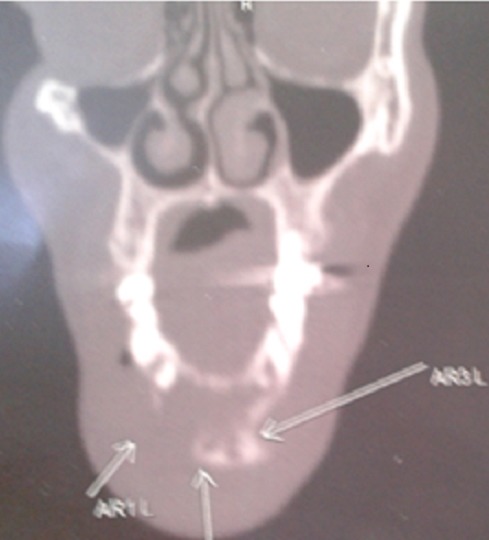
CT appearance of the chin recurrence
